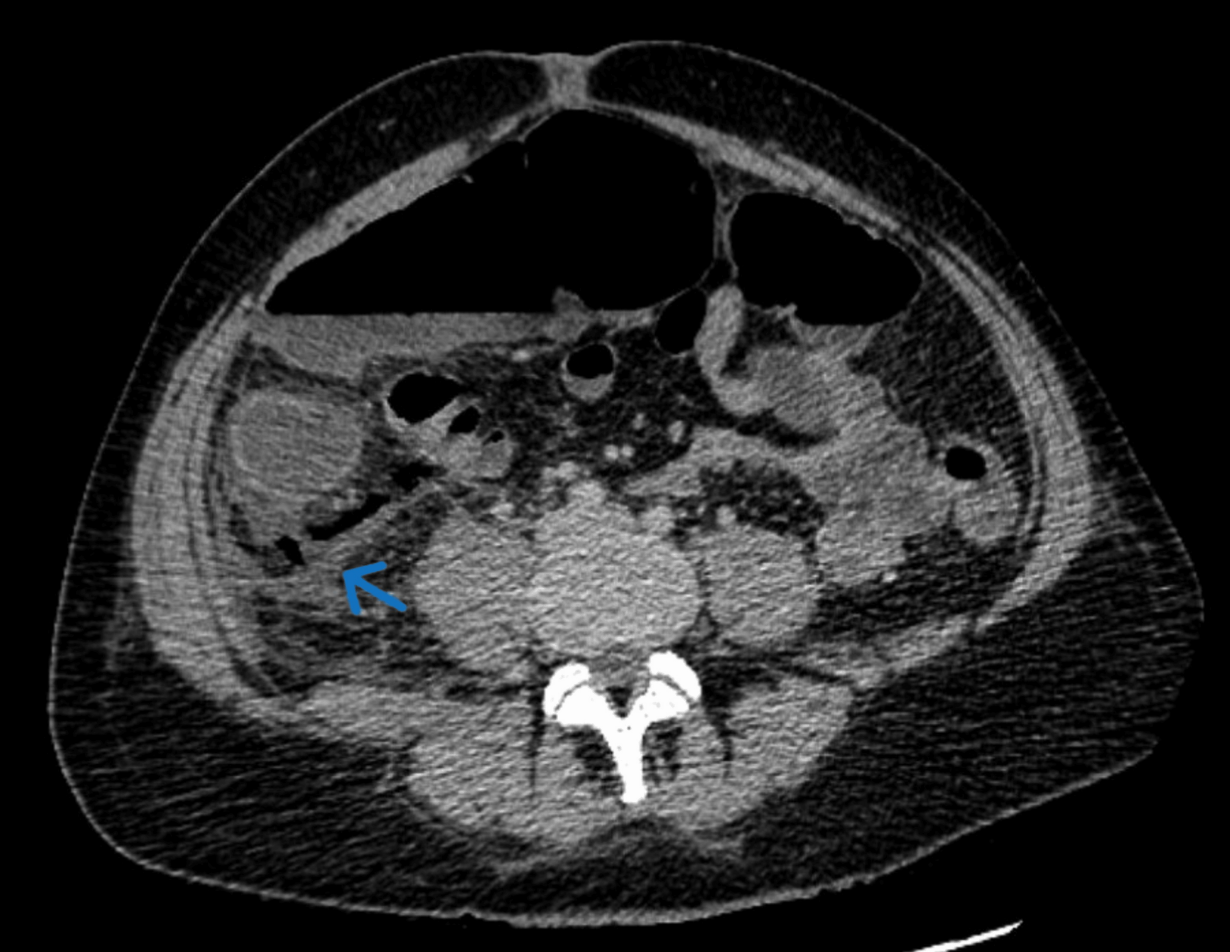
CT abdomen and pelvis (axial section) demonstrating free air and fluids in the right side of the abdomen (blue arrow)
